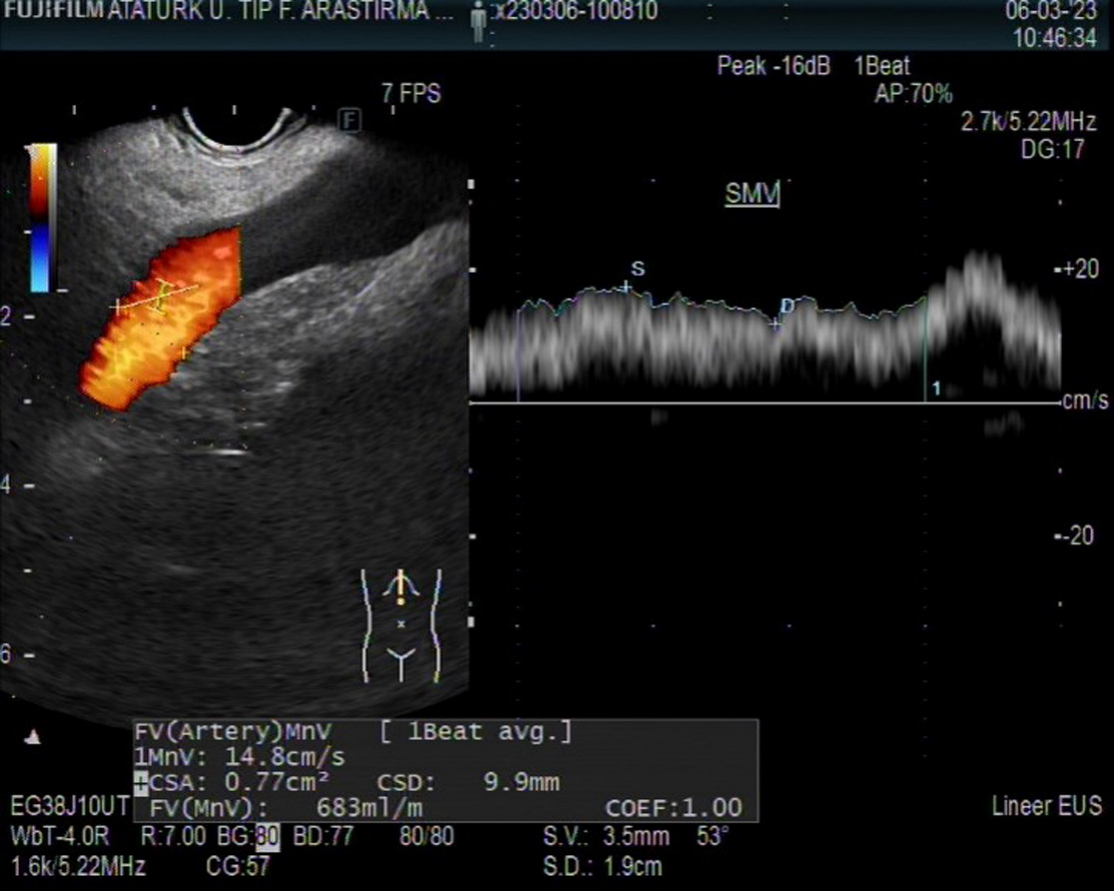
Superior mesenteric vein Doppler spectrum and flow velocity.
